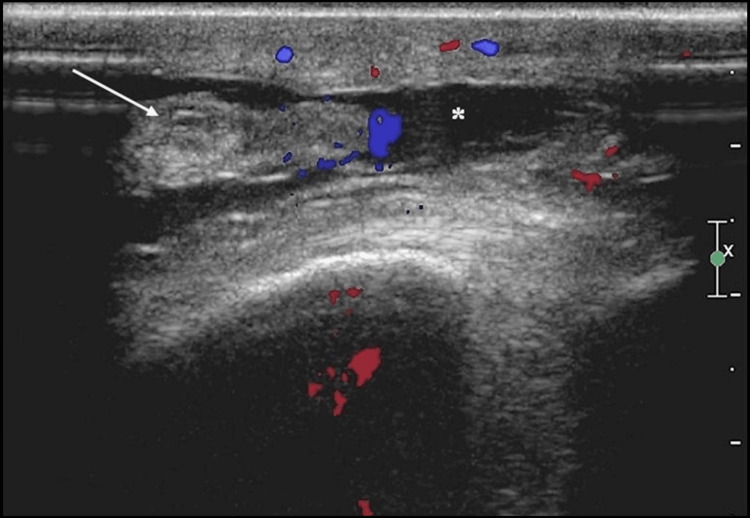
Doppler-ultrasound image of prepatellar soft tissue revealing a well-defined fluid collection (asterisk) within subcutaneous tissue containing some fat lobules (arrow) with no hyperemia.
